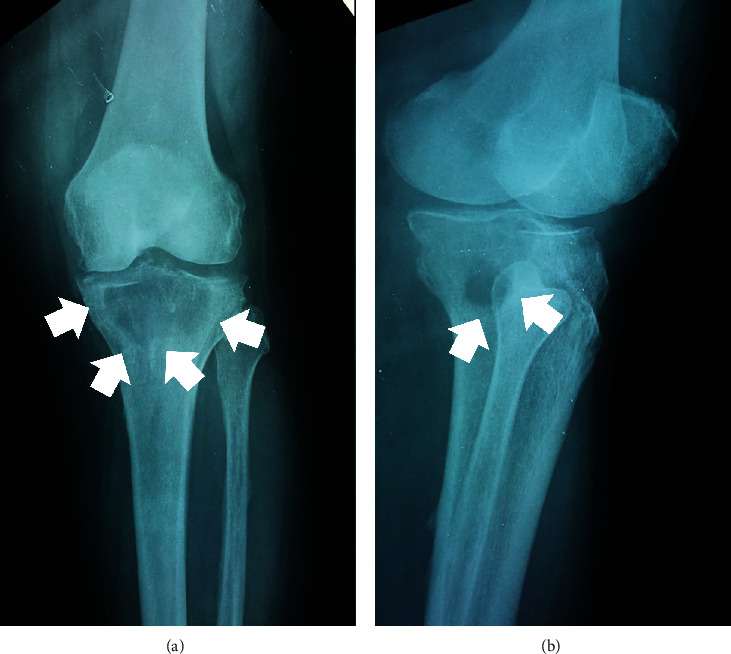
Preoperative radiograph ((a) anteroposterior, (b) lateral) of the left knee, depicting the existence of a large lytic lesion (white arrows) at the proximal tibia, extending into the popliteal cavity.
